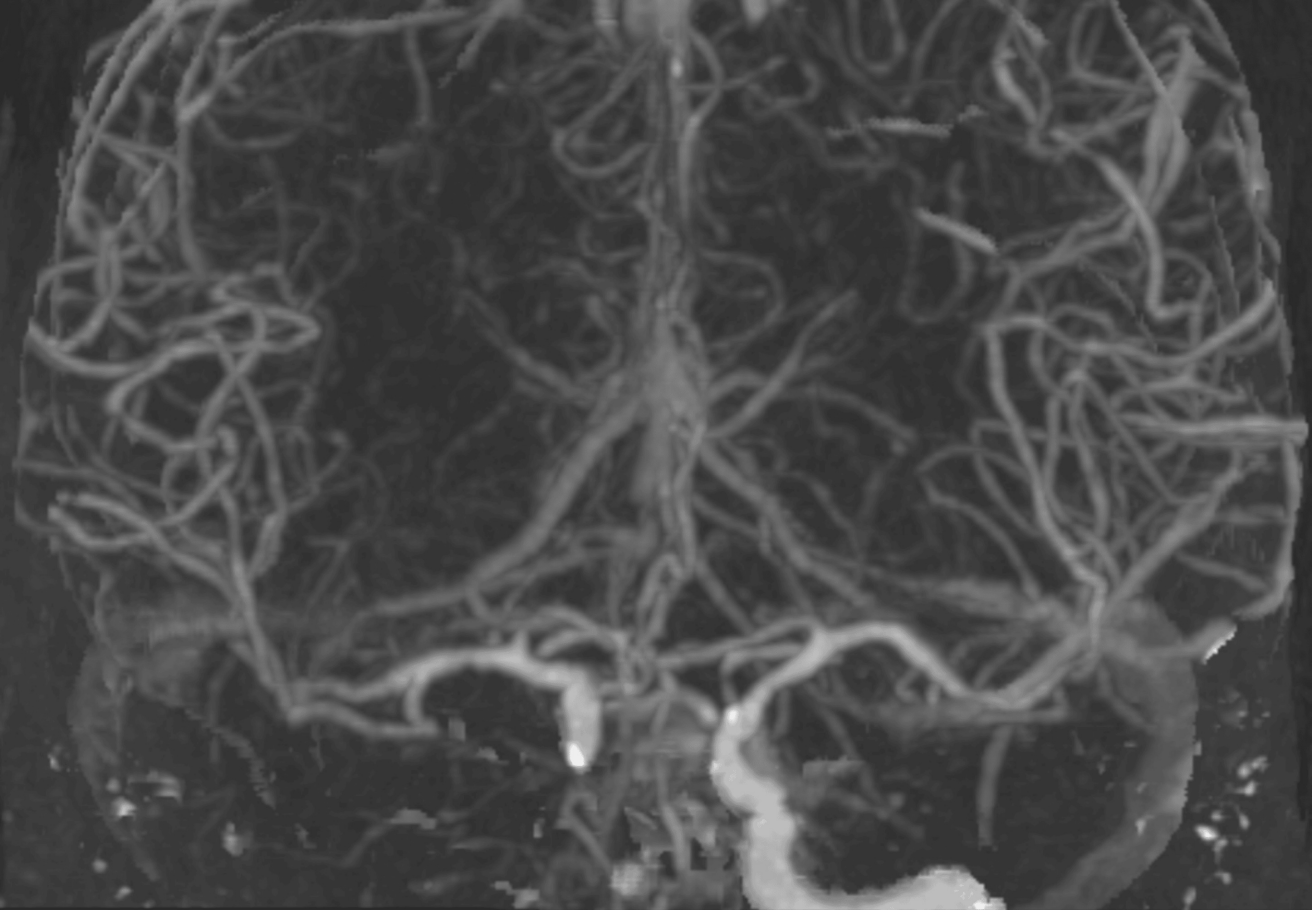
MIP from a CT angiogram of the head demonstrating the intracranial arterial circulation showing severe intracranial stenosis of the right internal carotid artery at the cavernous segment CT: computed tomography, MIP: maximum intensity projection
